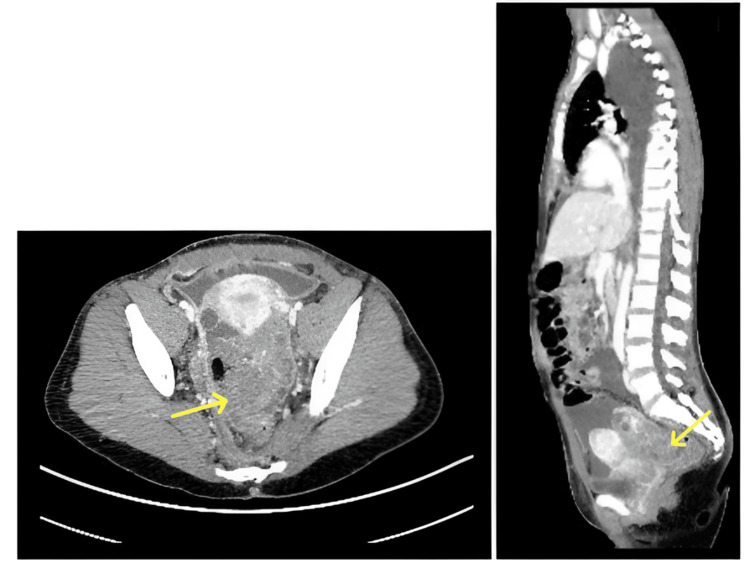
Preoperative CT image Preoperative CT showing a 6.4 cm x 8.2 cm predominantly soft tissue density lesion (denoted by a yellow arrow) with a small fatty component in the cul-de-sac.
